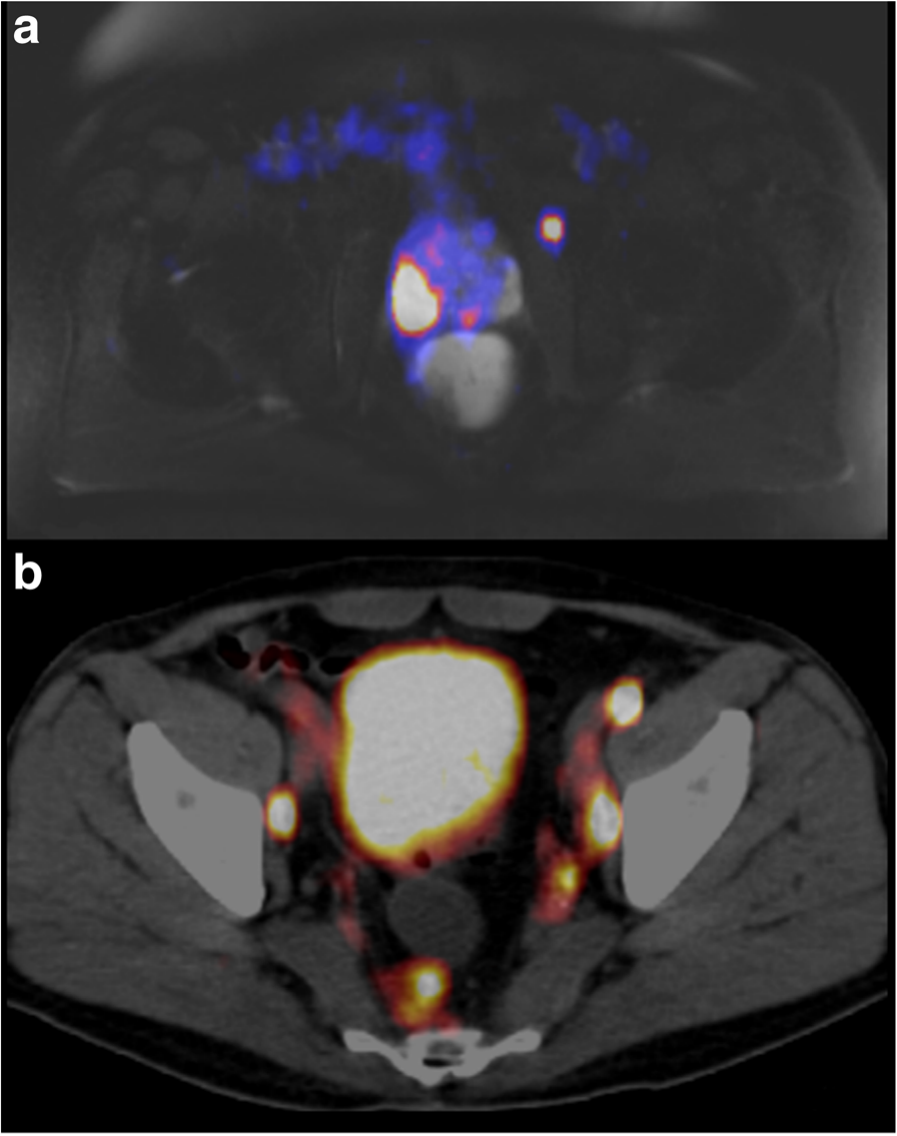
Images (a-b): lymphnodes on original ^68^Ga-PSMA-PET imaging slices: (a) uncommon lymphnode lateral of the M. obturatorius int. (compare image 2f) on a ^68^Ga-PSMA-PET-MRI, (b) several iliacal lymph nodes and one para-rectal lymph node (compare image 2d) on a ^68^Ga-PSMA-PET-CT
